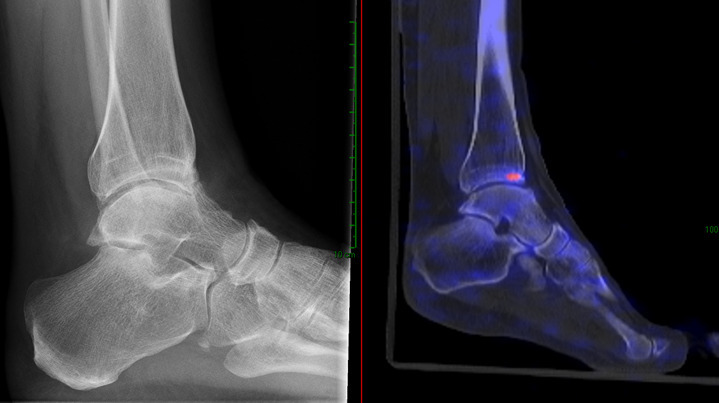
This patient (male of 64 years old) has a premedical history of excision of a chondral lesion and a corrective osteotomy for equines around 30 years ago. Since 5 years, there is a progressive pain on the lateral side-of the foot and midfoot. On examination, the foot is in slight varus but is correctable, The foot can dorsoflex no more than 5 degrees because of stiffness. There is pain when applying pressure over the talocrural joint, subtalar joint, and talonavicular joint. The X-ray showed mild OA of the talocrural joint and the talonavicular joint. The observer scored this case as talocrural, subtalar and talonavicular OA. After the SPECT/CT, the diagnosis changed in only talocrural OA. The suggested treatment plan was an orthopaedic shoe and did not changed after evaluating the SPECT/CT.
